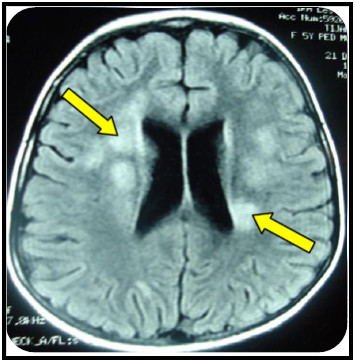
Brain MRI of the patient with juvenile form of MLD.
